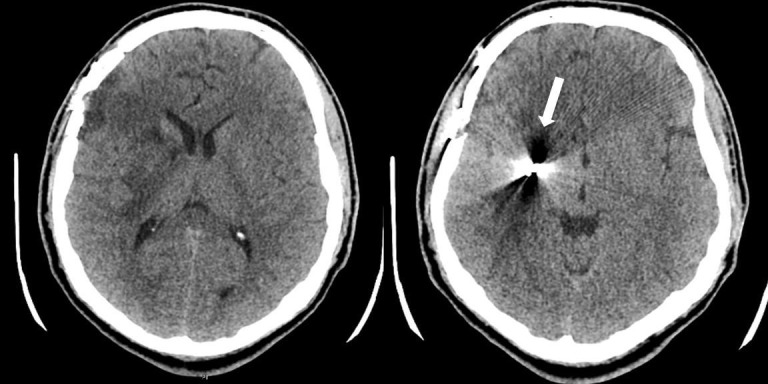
Post-operative computed tomography (CT) head without contrast revealing stable position of the retained high-velocity projectile adjacent to the right basal ganglia.
